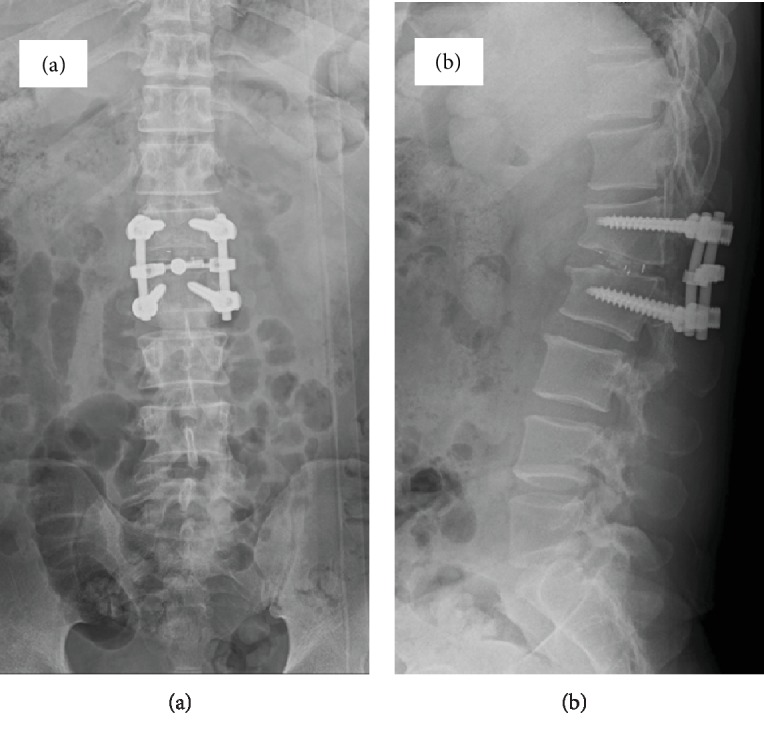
Postsurgery radiographs. Anteroposterior (a) and lateral (b) views, showing instrumented posterolateral interbody fusion at L1-2.
